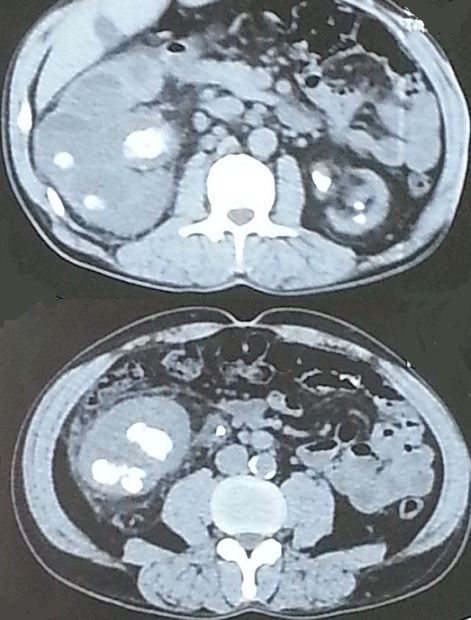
CT showing a right hydronephrosis and hypotrophic left kidney secondary to bilateral renal pelvis and caliceal stones
